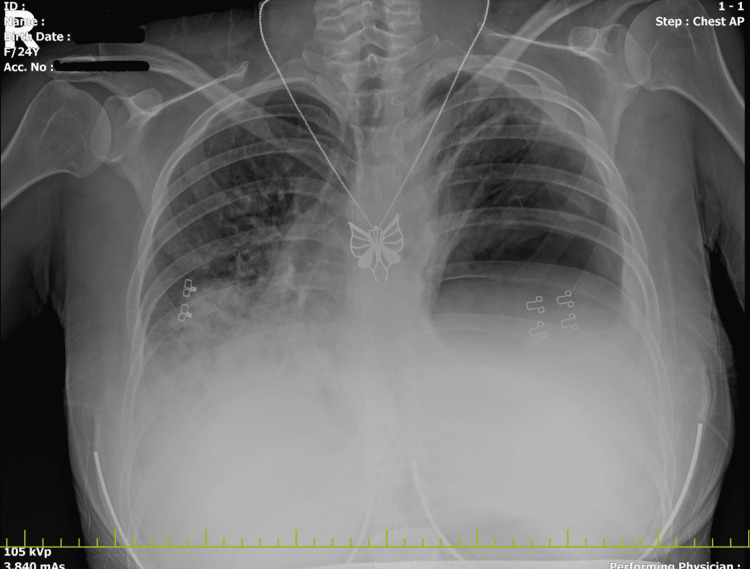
Chest X-ray Huge cystic lesion with an air-fluid level located in the lower and mid-zone of the left lung causing mediastinal shift.
